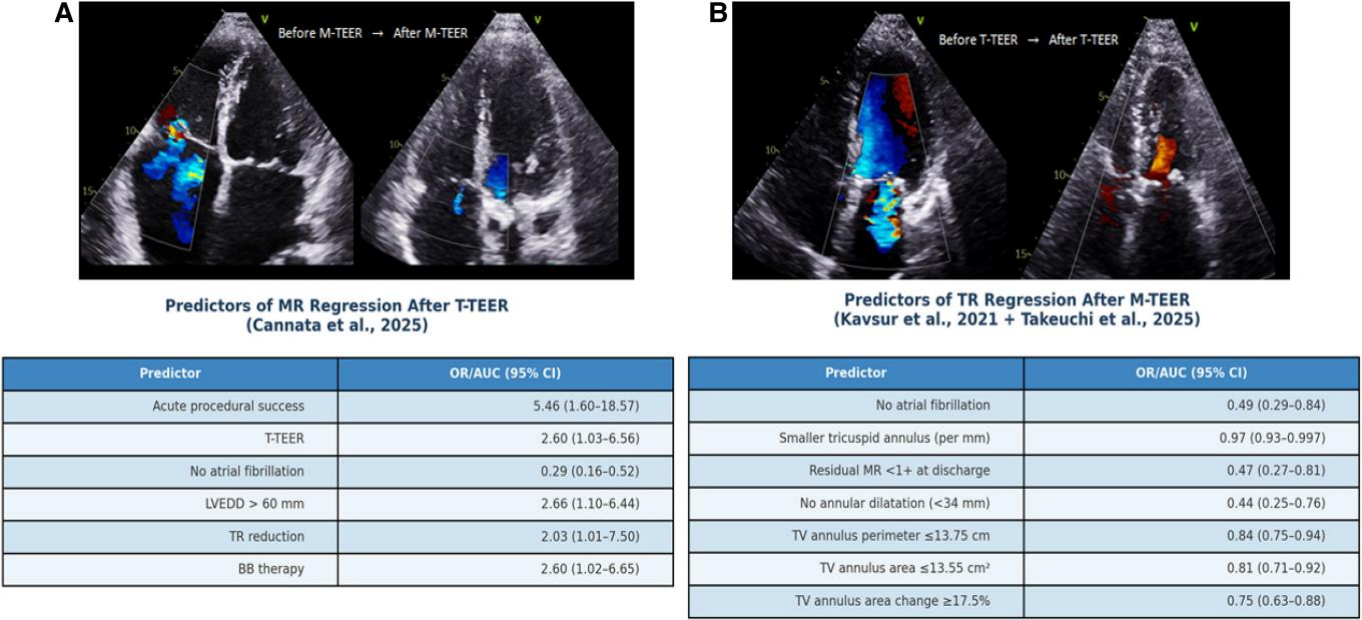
Echocardiographic improvement and independent predictors of mitral and tricuspid regurgitation regression following TEER. Top: colour Doppler images before and after M-TEER (left) and T-TEER (right). Bottom: predictors of regurgitation regression from multivariable models.^25,26,27^ All predictors shown were statistically significant (*P* < 0.05). OR, odds ratio; AUC, area under the curve; CI, confidence interval.
